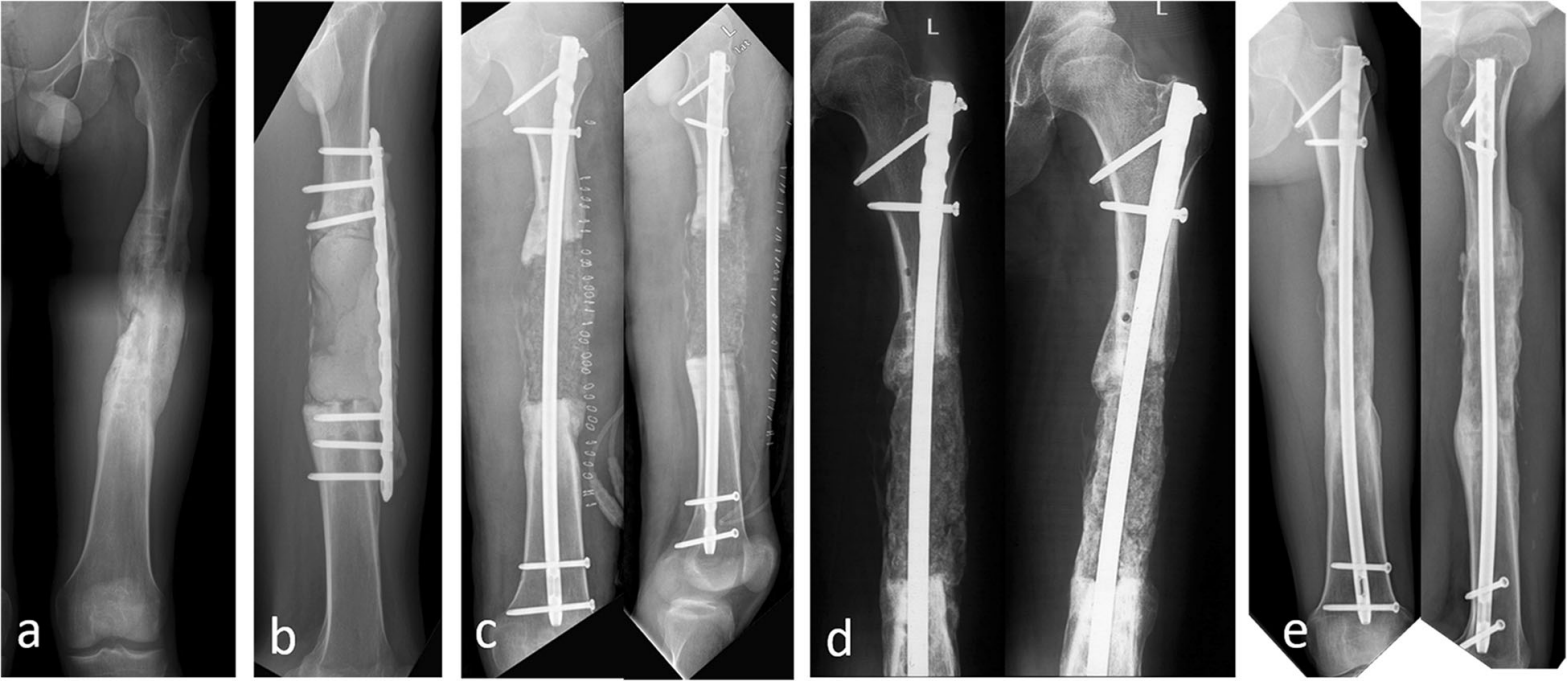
Twenty-one-year-old male suffered from bone infection of the left femur for 2 years. The result of bacterial culture during operation was *Enterobacter cloacae*. a X-ray shows bone destruction. b Resection of the lesion; antibiotic cement filled the defect and wrapped the plate. c Bone graft after 8 weeks. d Callus growth was observed after 3 months. e Bone union after 24 months
